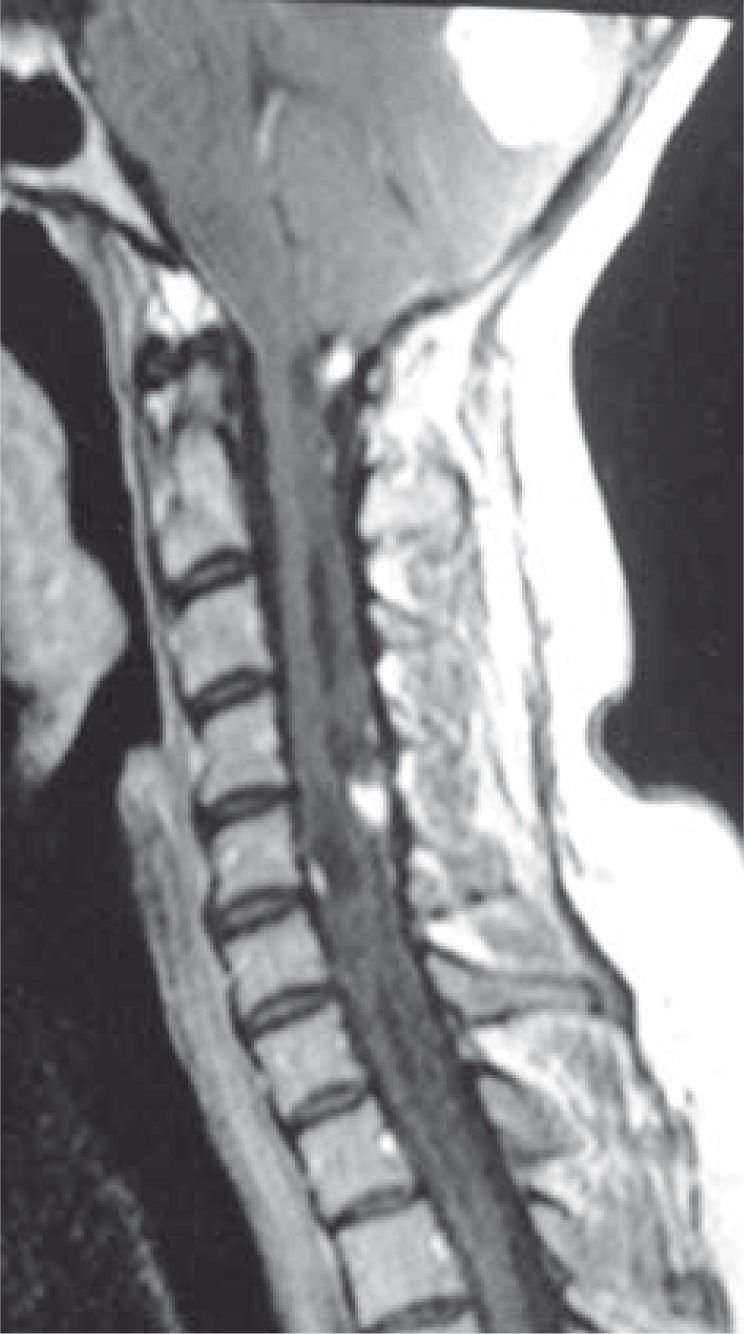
Sagittal Axial T2 weighted MR image shows multiple cysts in kidney and pancreas.
